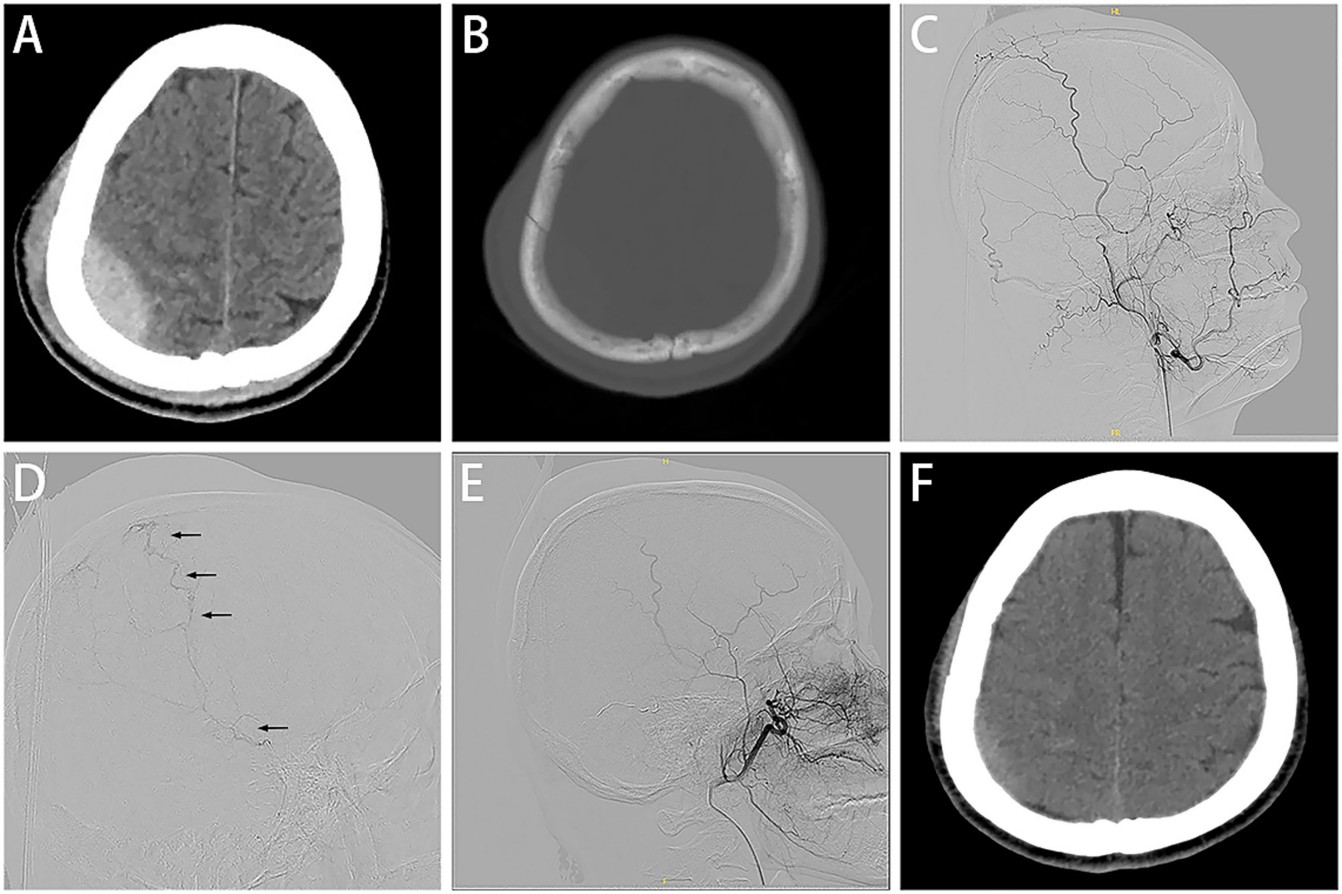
Case 2 representative images. Initial CT scans revealed acute EDH in the right parietal region adjacent to fracture (A,B). Ipsilateral ECA angiography shows no abnormal findings (C). Super-selective MMA angiography via a microcatheter (D) shows abnormal dilation of branch vessels and extravasation of the contrast medium (black arrow). Embolization of the lesion (E). Follow-up CT represented a marked decrease of the EDH (F).
